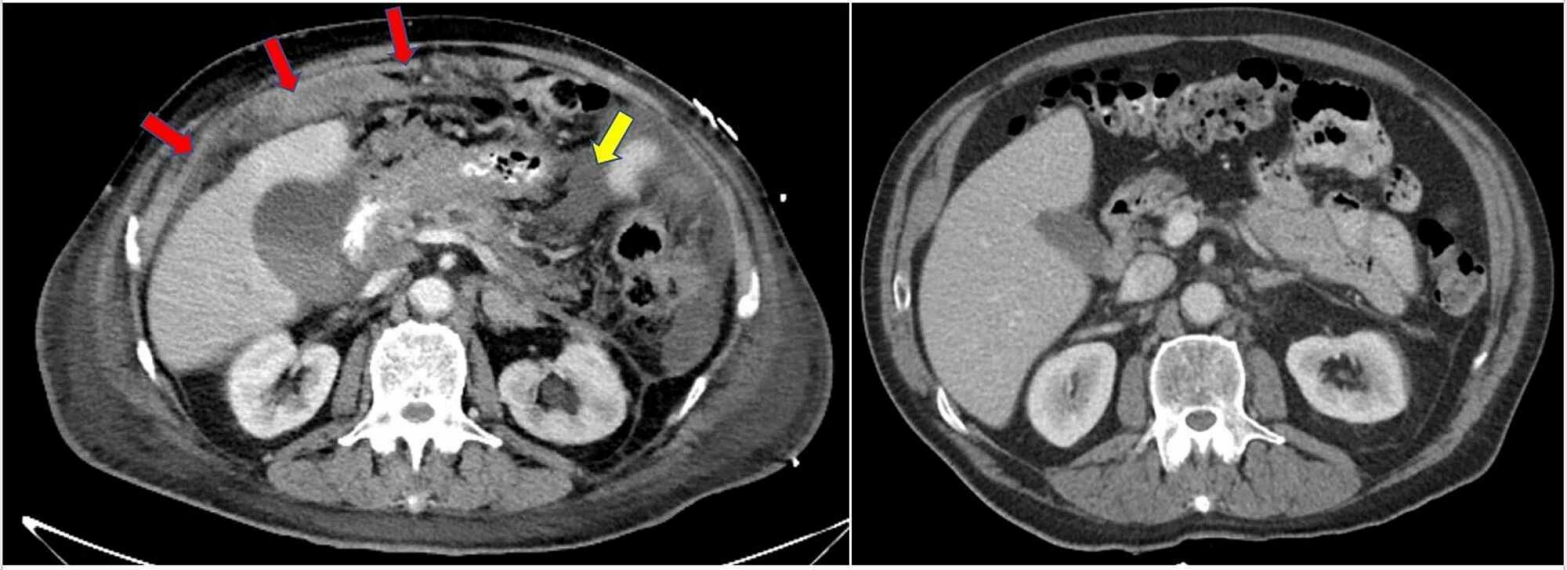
CT scan image of the abdomen (left) showing new omental carcinomatosis (red arrows) and ascites (yellow arrow) compared to the CT scan done three months prior (right). CT, computerized tomography
